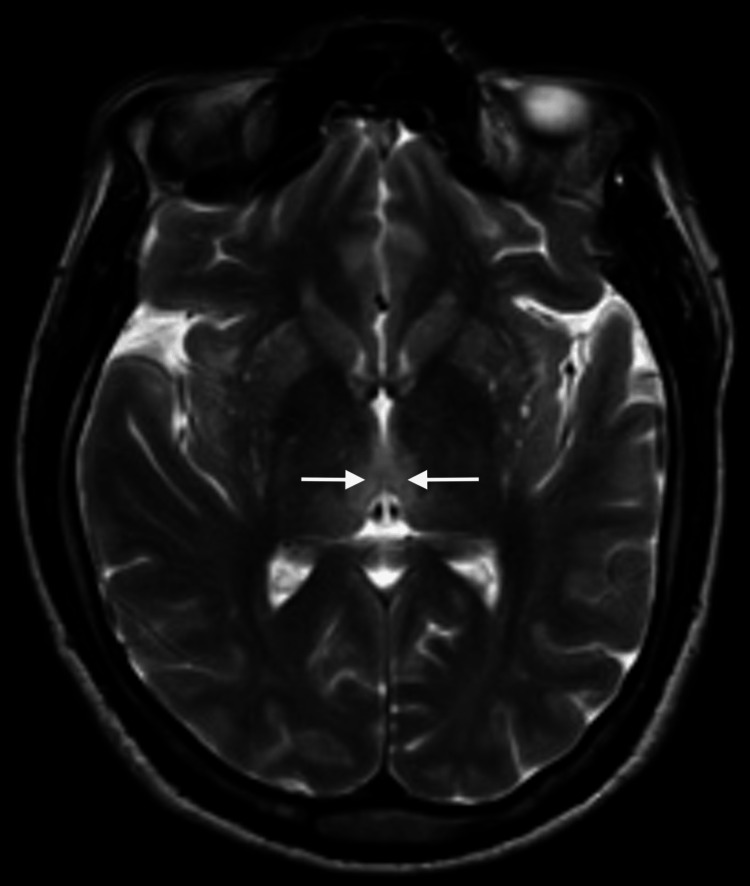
Contrast-enhanced MRI: An initial study. Axial T2 thalami. Bilateral and symmetrical hyperintensity of the dorsomedial aspect of the thalami is observed on T2-weighted images (arrows).
